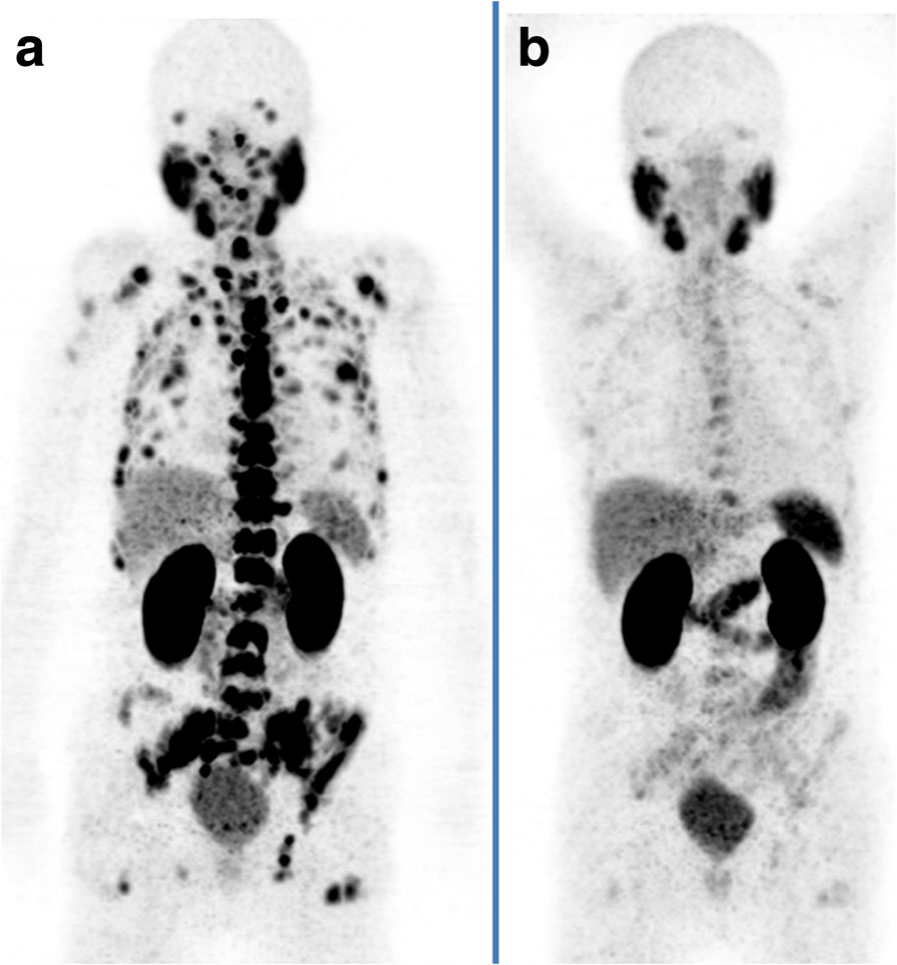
A 83-year-old patient with castration-resistant prostate cancer (Gleason-Score:9) and an increasing prostate-specific antigen (PSA) level. He had a history of prostatectomy and radiation therapy of prostate bed. The 68 Ga-PSMA PET scan showed a diffuse bone and bone marrow involvement (a). The PSA and ALP levels prior to the first cycle of Lu-PSMA therapy were 261 ng/ml and 659 U/l, respectively. The patient received 2 cycles of Lu-PSMA and the PSA level decreased continuously during cycles from 261 to 9.0 ng/ml (8 weeks after the second cycle). The ALP showed also a decreasing value from 659 to 81 U/l (8 weeks after the second cycle). The PSMA-PET (b) 8 weeks after the second cycle showed a significant response with significant regression of PSMA
